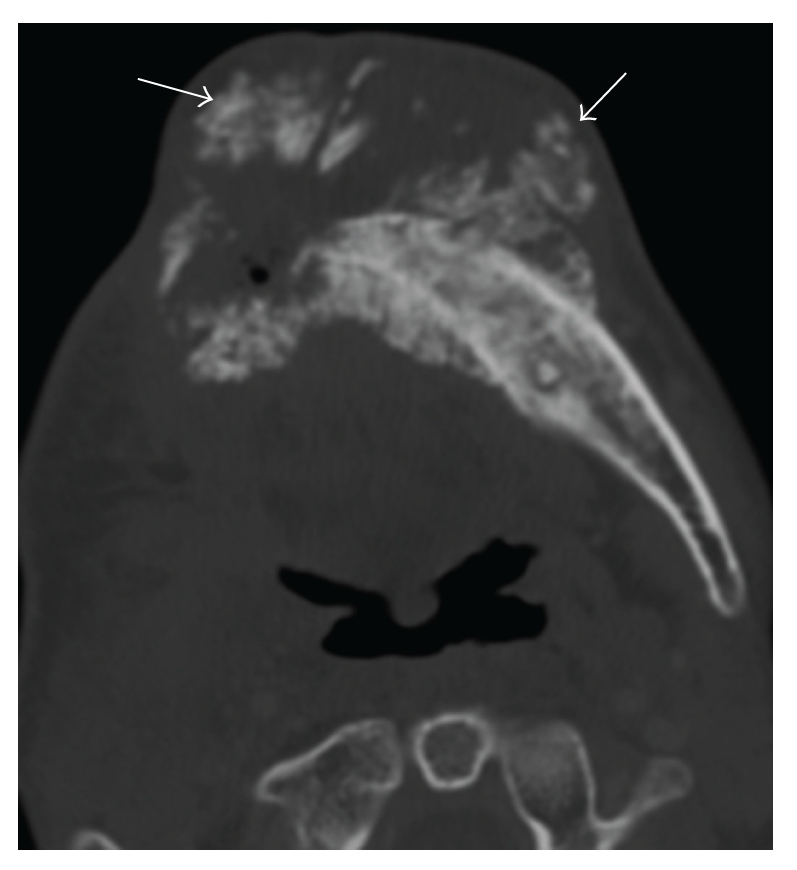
Radiation-associated osteosarcoma of the mandible: (a) Axial contrast-enhanced CT of the mandible (bone window) shows an osteoid matrix (arrow) within the tumor.
